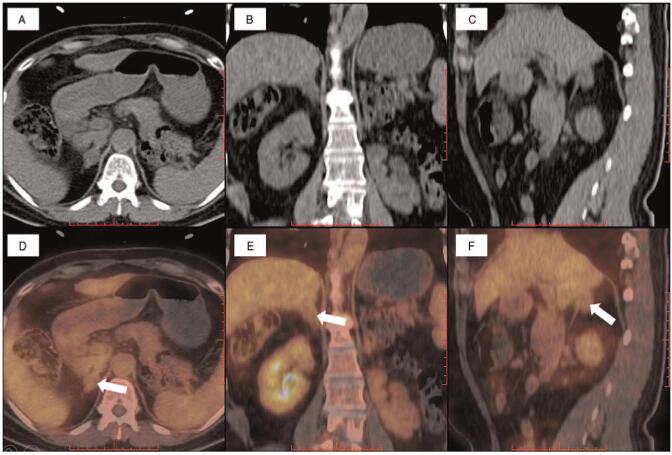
PET/CT scan showed high radio uptake mass on the right adrenal gland (white arrows). (A&D) Axial PET/CT images: CT image and fusion image. (B&E) Coronal PET/CT images: CT image and fusion image. (C&F) Sagittal PET/CT images: CT image and fusion image.
